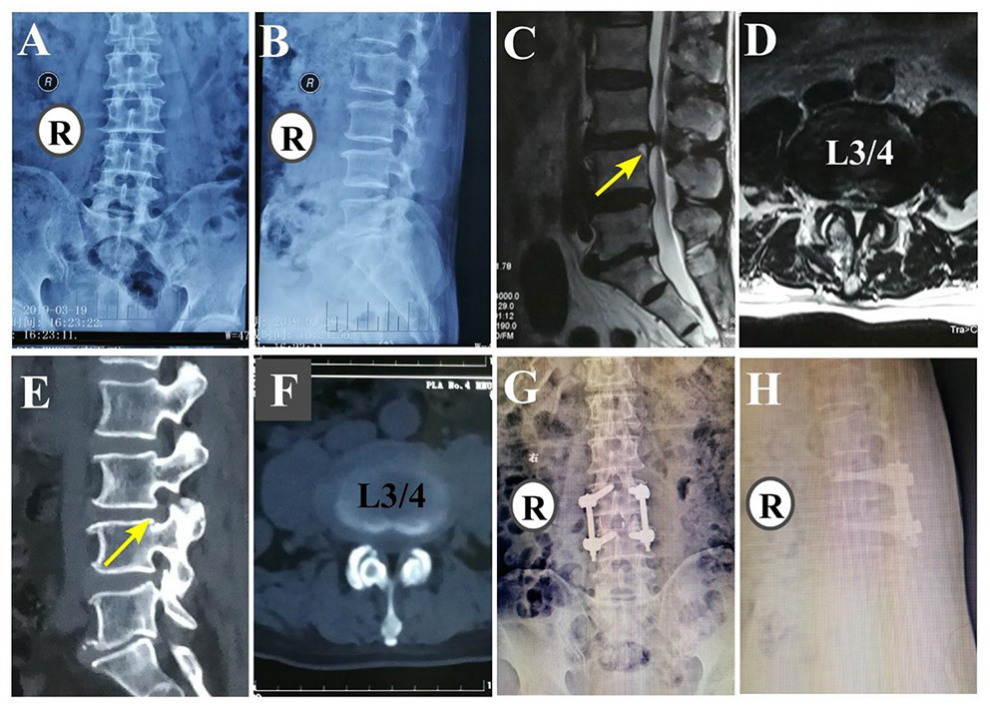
A 79-year-old man with low back pain and weakness of both lower extremities with OLIF (L3/4). (A,B) Preoperative anteroposterior and lateral fluoroscopy. (C,D) Preoperative sagittal and axial MRI. (E,F) Preoperative sagittal and axial CT (G,H) Postoperative anteroposterior and lateral fluoroscopy.
